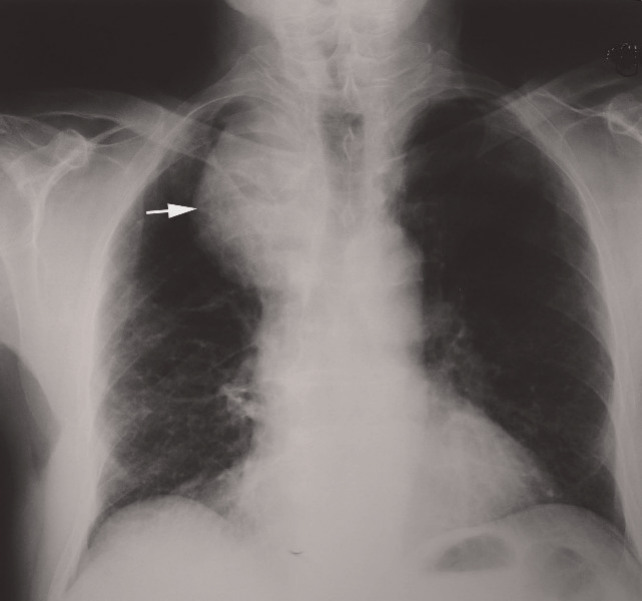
Posteroanterior chest radiograph shows a mass (arrows) in the mediastinum with deviation of the trachea.
